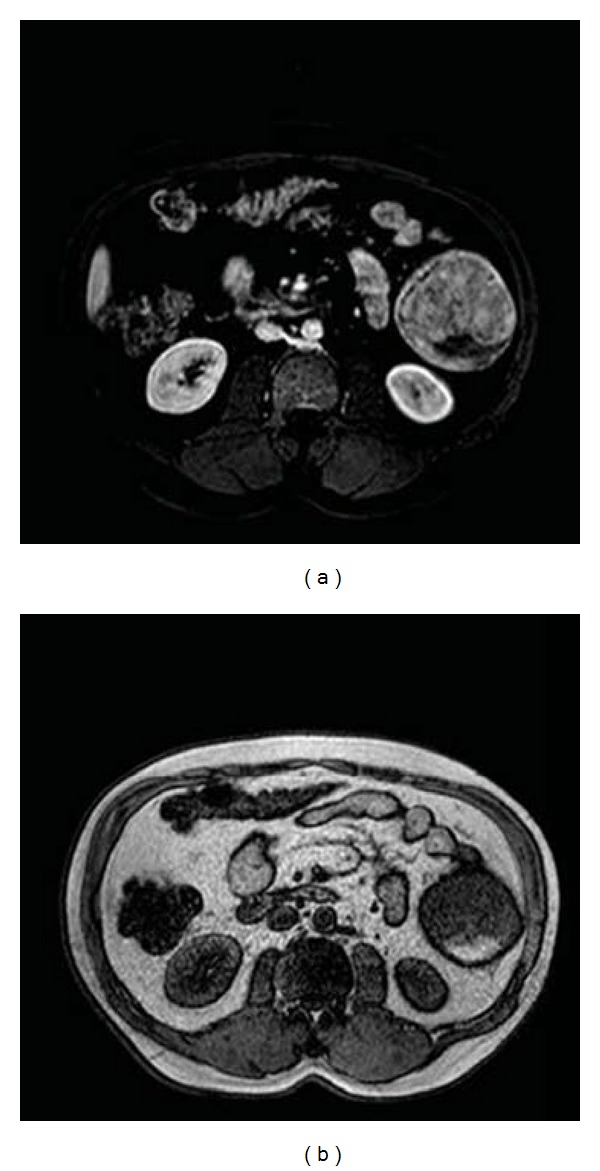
(a) Intense contrast enhancement of splenic mass. No enhancement detected at the inferior side suggesting bleeding. (b) MRI: regular-bordered mass with heterogenic hyperintense area at inferior pole on axial T1 weight pulse sequence.
